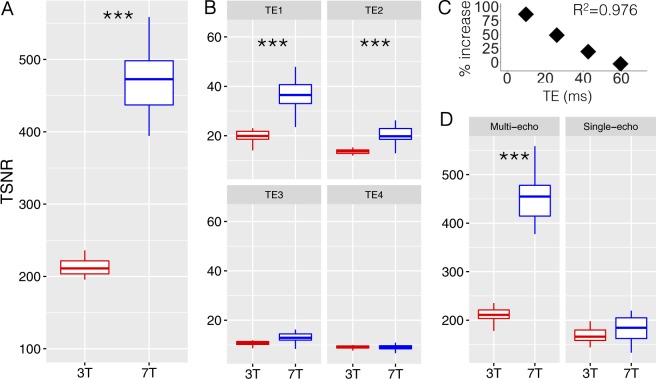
Higher temporal signal to noise ratio (TSNR) with ultra-high field 7-Tesla (7 T) functional magnetic resonance imaging (MRI) scales with echo time (TE) and is boosted by multi-echo based denoising. a TSNR plotted for whole brain at 3-Tesla (3 T) and 7 T. b TSNR plotted for 7 T at 3 T for 4 different TE’s (TE1 = shortest, TE4 = longest). c Percent increase in TSNR at 7 T compared to 3 T scales with TE (Linear fit, adjusted *R*^2^ = 0.976, *p* = 0.008). d TSNR plotted for whole brain after multi-echo acquisition and denoising and traditional single-echo acquisition and denoising. p* ≤ 0.001
